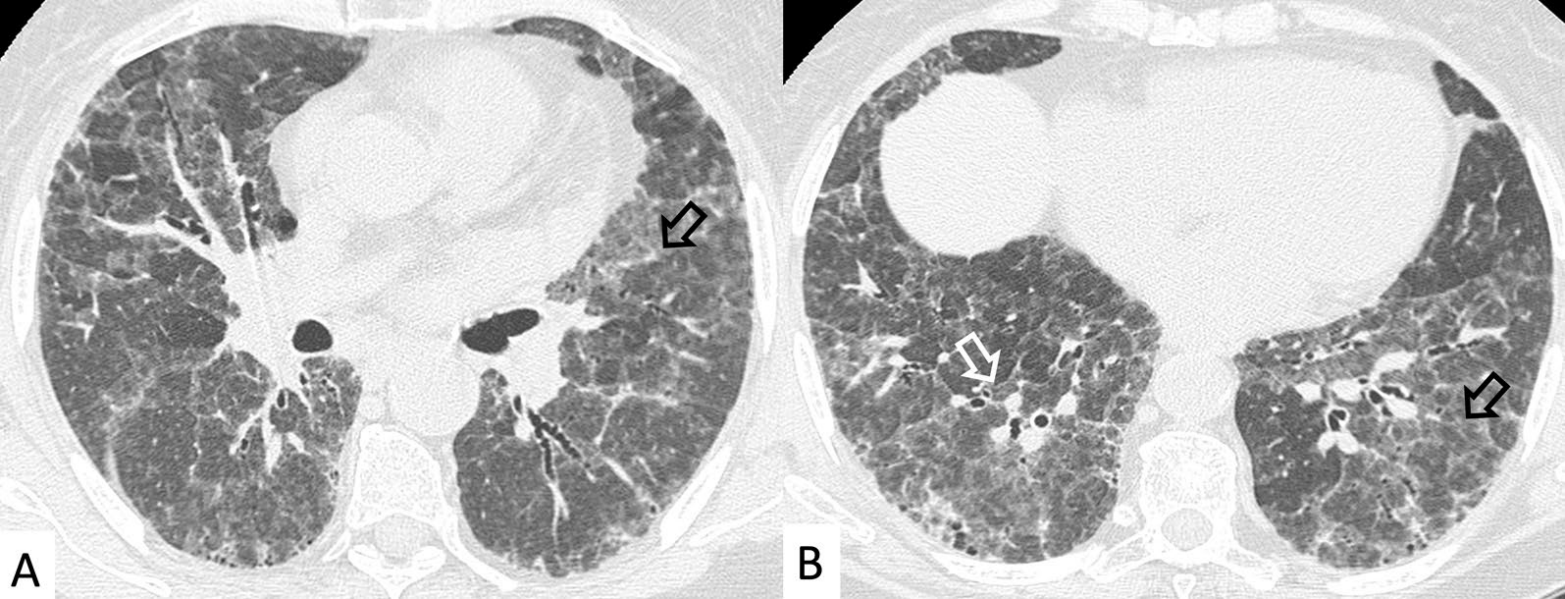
A NSIP pattern in a female patient with diagnosis of MCTD. HRCT show ground-glass areas (white and black empty arrows) in figures A and B. Reticulations and small cystic areas are also evident, in this fibrosing pattern
